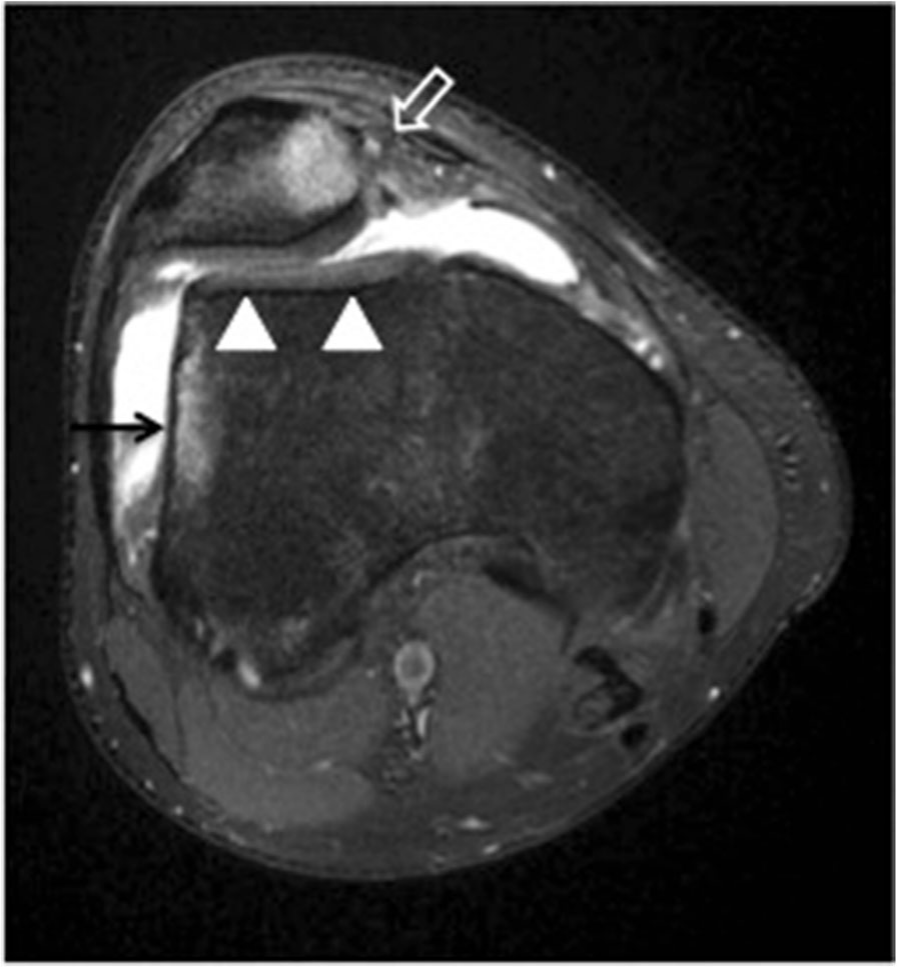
Transient lateral patellar dislocation. Axial PDFS MR image showing MPFL disruption (open arrow) and trochlear dysplasia (arrowheads). There is edema of the medial patella and of the lateral femoral condyle (arrow), consistent with bone contusion due to recent lateral patellar dislocation
